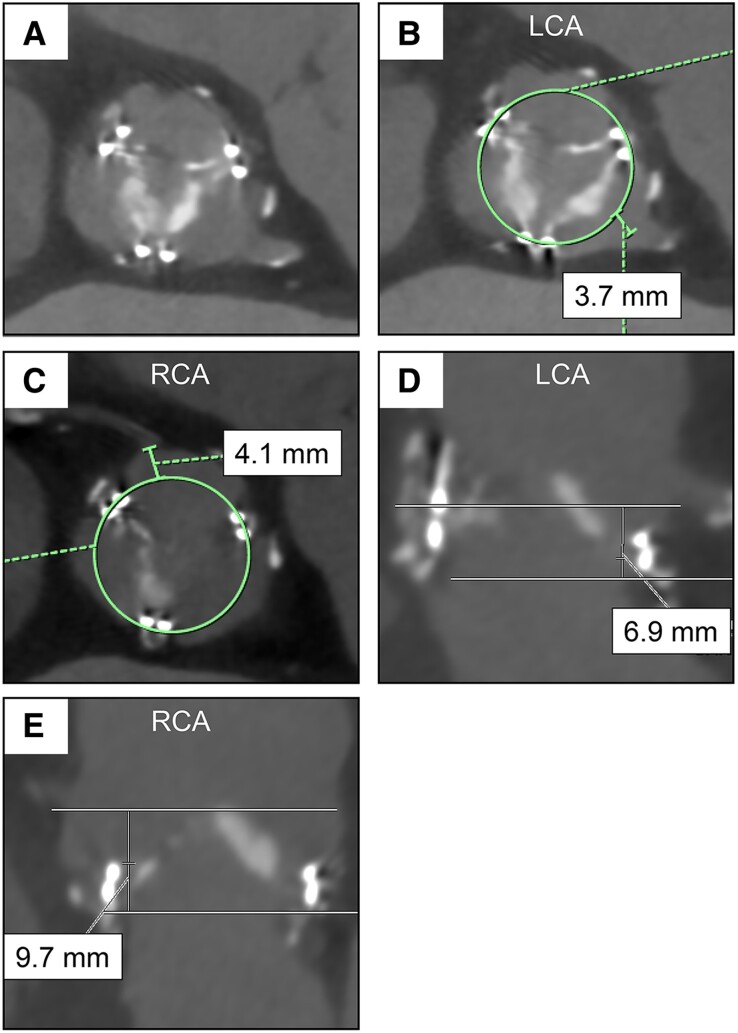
Preprocedural computed tomography. (*A*, *B*) Severely calcified and thickened leaflet. (*C*) Virtual transcatheter heart valve to left coronary ostium distance. (*D*) Virtual transcatheter heart valve to right coronary ostium distance. (*E*) Left coronary height. (*F*) Right coronary height. LCA, left coronary artery; RCA, right coronary artery.
